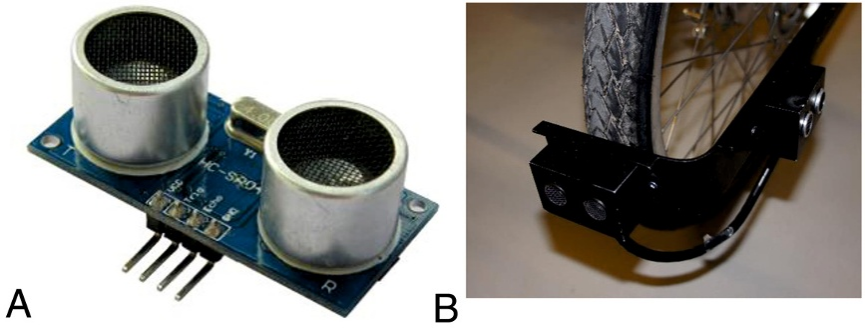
Distance detection design. (A) Ultrasonic sensor; (B) Retainer with mounted distance measurement unit.
